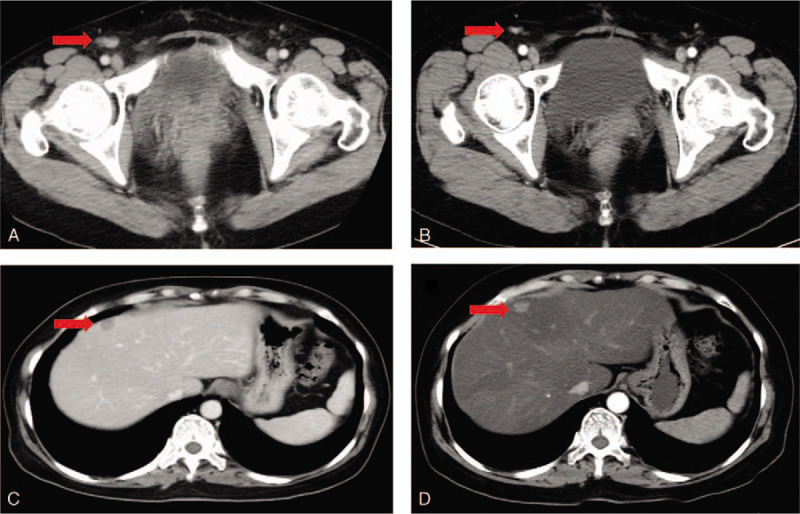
CT image showing metastases before and during the treatment of anlotinib plus etoposide. CT image showing (A) lymph nodes metastases in the right inguinal region (C) liver metastases before treatment of anlotinib plus etoposide (October 9, 2018). CT image showing (B) smaller lymph nodes metastases in the right inguinal region (D) similar liver metastases after eleven weeks of anlotinib plus etoposide treatment (December 24, 2018).
